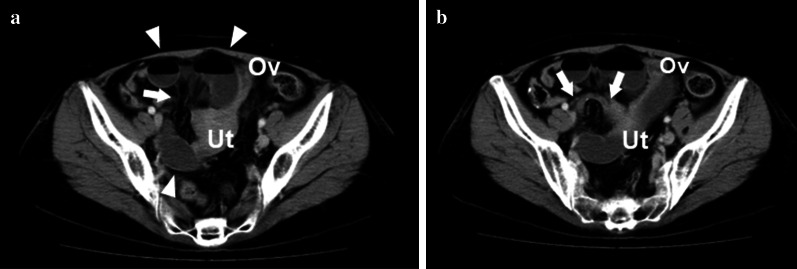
Contrast-enhanced computed tomography (CT) findings. Small-bowel loops (arrowheads) were dilated in the vicinity of the uterus and the mesentery converged at the right side of the uterus (arrow). The uterine showed counterclockwise rotation (a). The next slice of A revealed a thick band (arrows) interpolated in the convergence of the mesentery (b). Ut and Ov indicate uterine and left ovary, respectively
